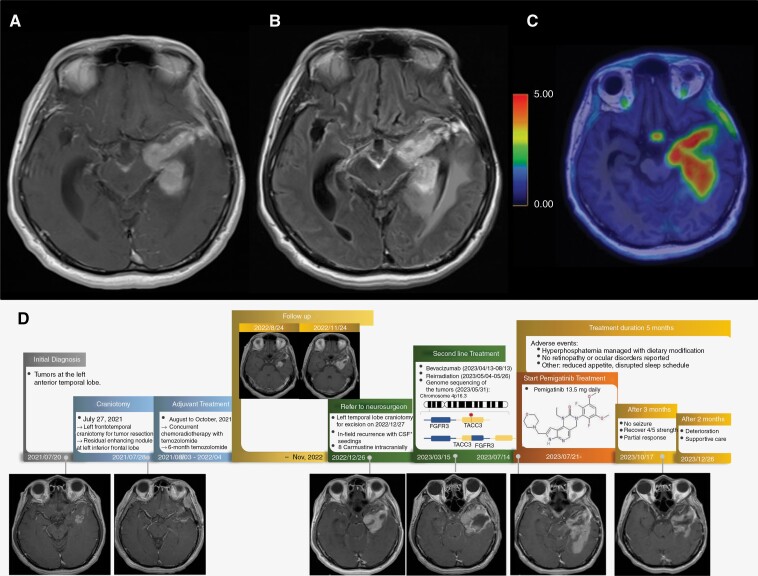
The enhancement in the left temporal lobe to the insula area with leptomeningeal seeding on the axial view of the T1-weighted with contrast (A) and T2-weighted fluid-attenuated inversion recovery with contrast (B) brain magnetic resonance imaging at recurrence before surgery. The axial view of an 18F-Fluciclovine amino acid positron emission tomography (C). Imaging series and treatment course of the patient (D).
